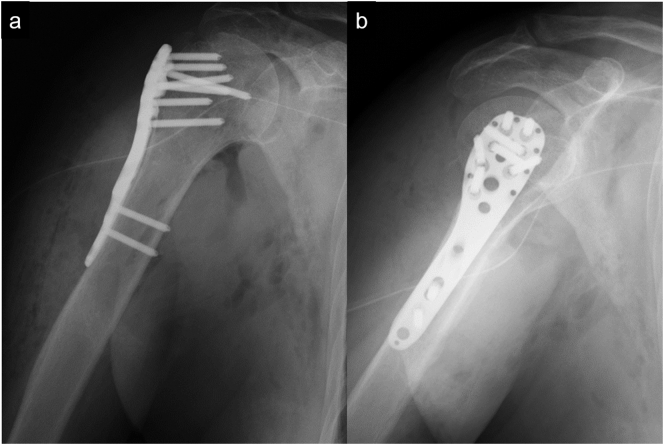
On postoperative plain radiographs, the glenohumeral joint was reduced, and the greater tuberosity was also fixed.
